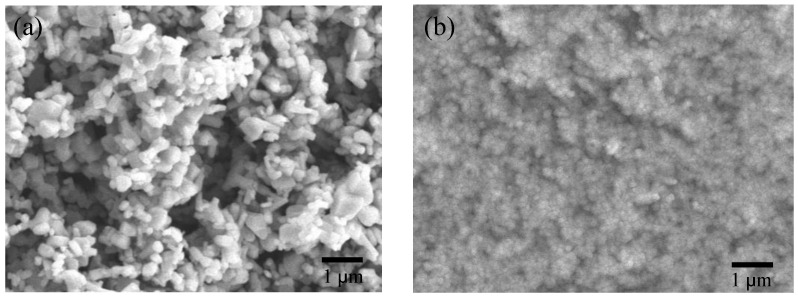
Top view SEM images of (a) origin-ZnO powder and (b) ZnO powder after ultrasonic grinding.
